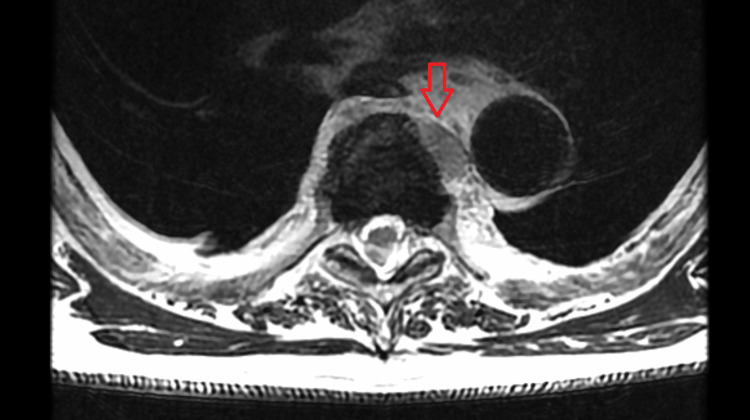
MRI of the dorso-lumbar spine showing a tumor mass in the T5 vertebra
